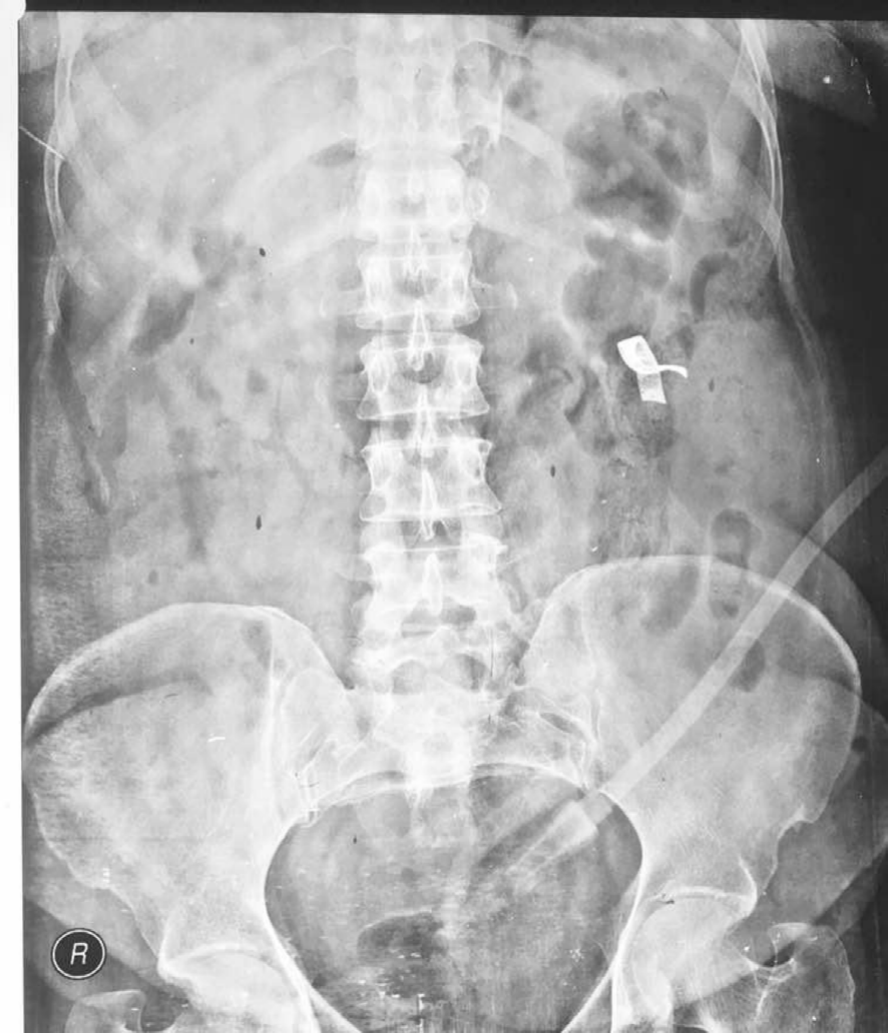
X-ray at 6 hour showing gauze in descedning colon.
